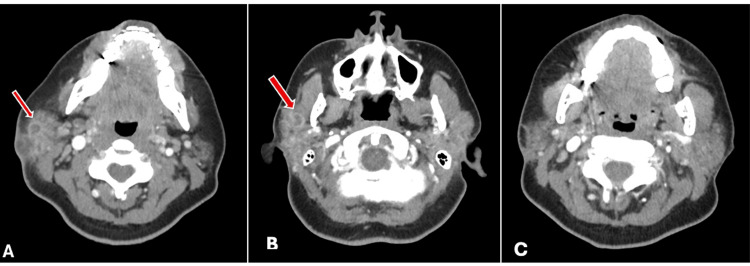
CT soft tissue neck scan showing (A) the initial rim-enhancing infection pre-treatment, (B) recurrence of symptoms and radiologic findings at five months, and (C) post-treatment following antibiotic therapy and superficial parotidectomy.
